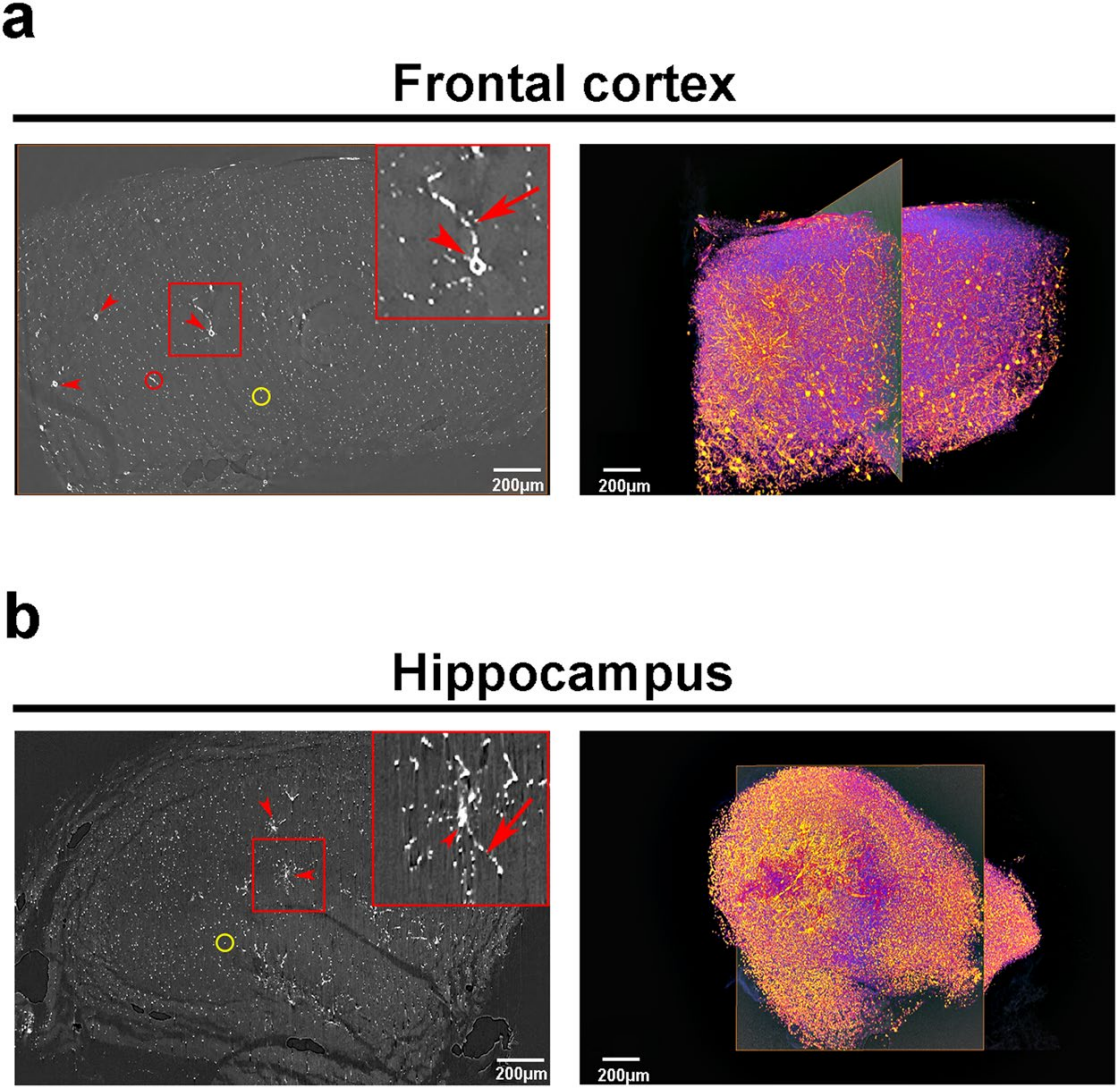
Synchrotron X-ray microtomography of mercury-impregnated neurons allows virtual slicing and 3D rendering of whole brain structures. Reconstructed slice (left panel) and 3D image rendering (right panel) of a control frontal cortex (a) and hippocampus (b). Rectangles in (a) and (b) highlights, respectively, a single cortical or hippocampal neuron. Arrowheads: neuron cell bodies; arrows: neurites; red circles: longitudinal virtual slicing of a neurite; yellow circles: transversal virtual slicing of a neurite.
